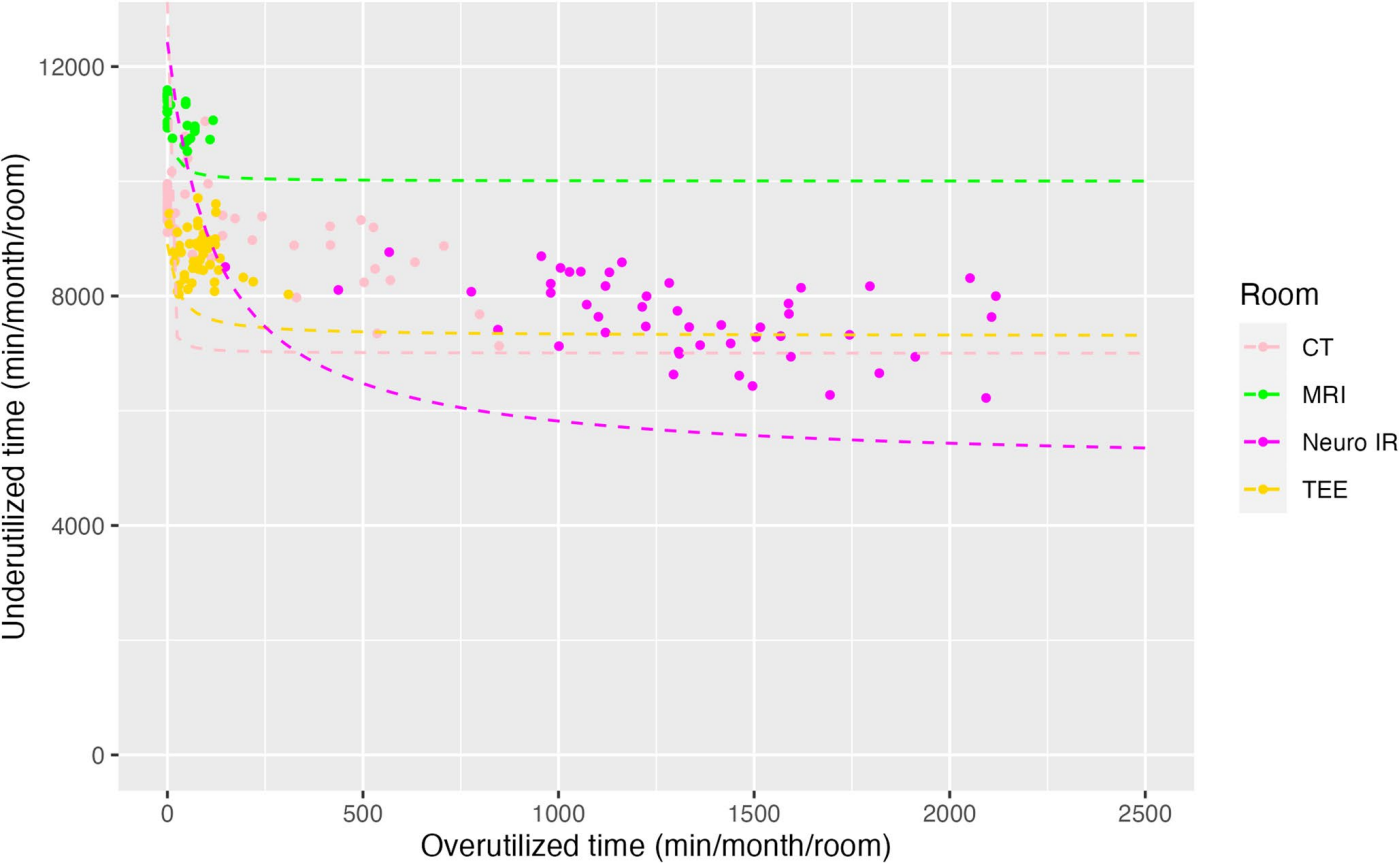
Performance frontiers of inefficient, sporadically utilized anesthesia locations consistently demonstrate a large amount of both under- and over-utilized minutes. CT, computed tomography; IR, interventional radiology; MRI, magnetic resonance imaging; TEE, transesophageal echocardiography
